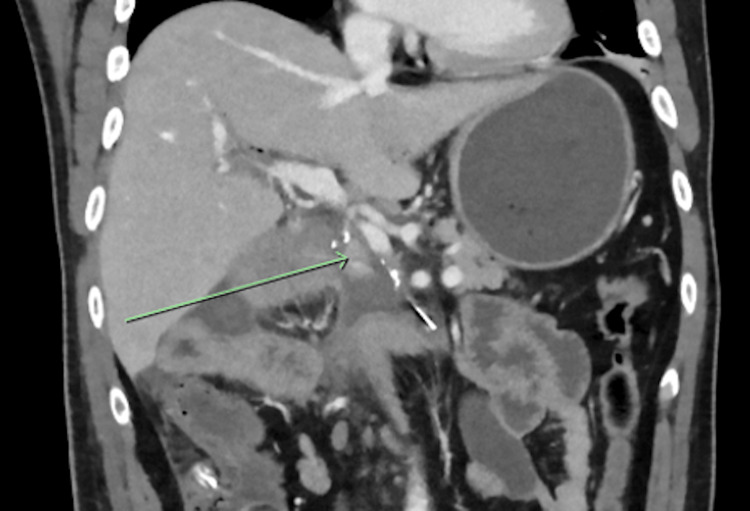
CT imaging day 8 postoperatively for a suspected acute intra-abdominal haemorrhage (June 2020) Coronal CT image with portal venous contrast enhancement demonstrating pooling of contrast (arrow) inferior to the gastroduodenal artery stump. CT, computed tomography.
